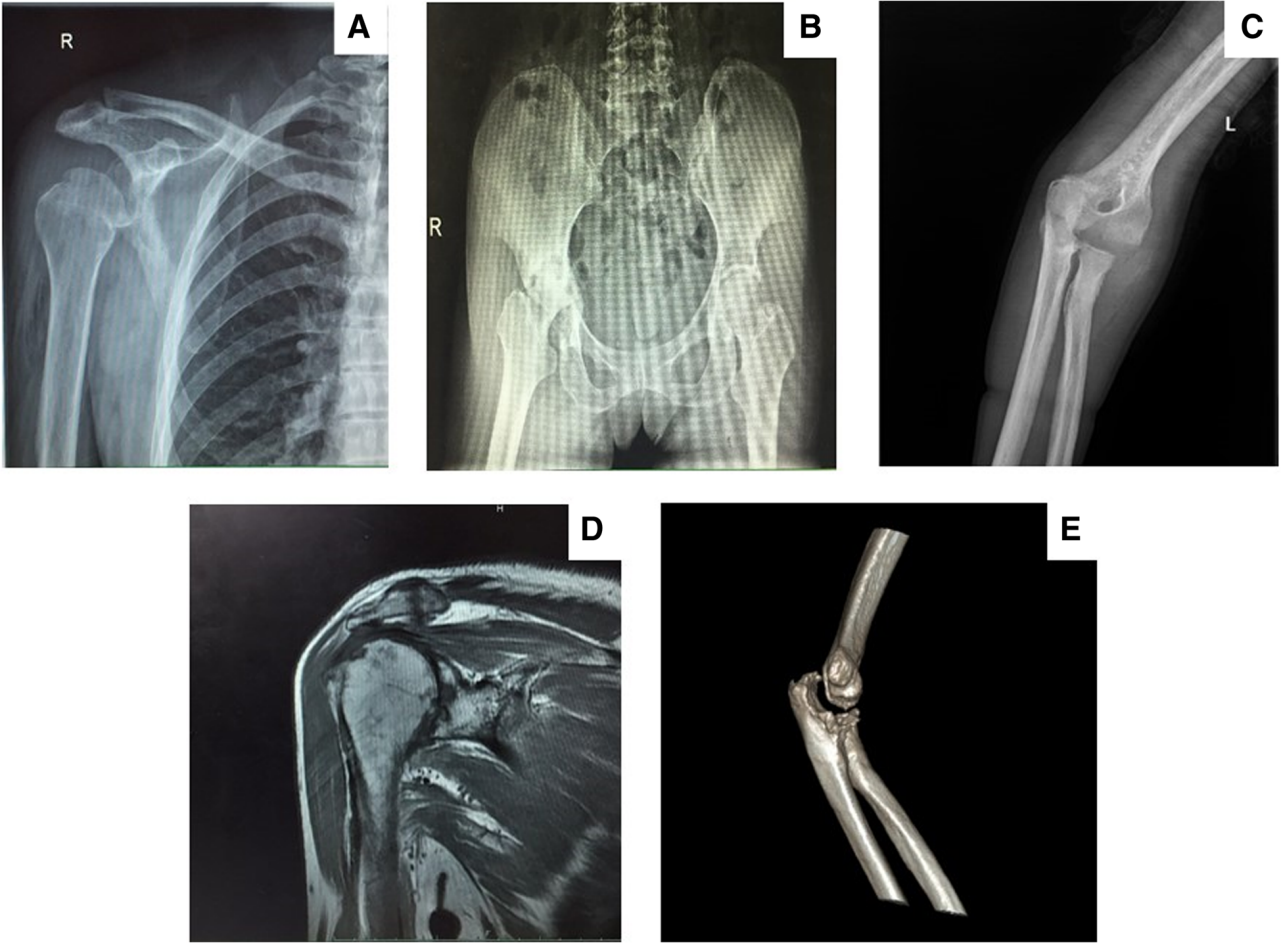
X-ray, CT and MR of different peripheral bone and joint tuberculosis. a X-ray of shoulder TB; b X-ray of hip TB; c X-ray of elbow TB; d MR of shoulder TB; e CT of elbow TB
